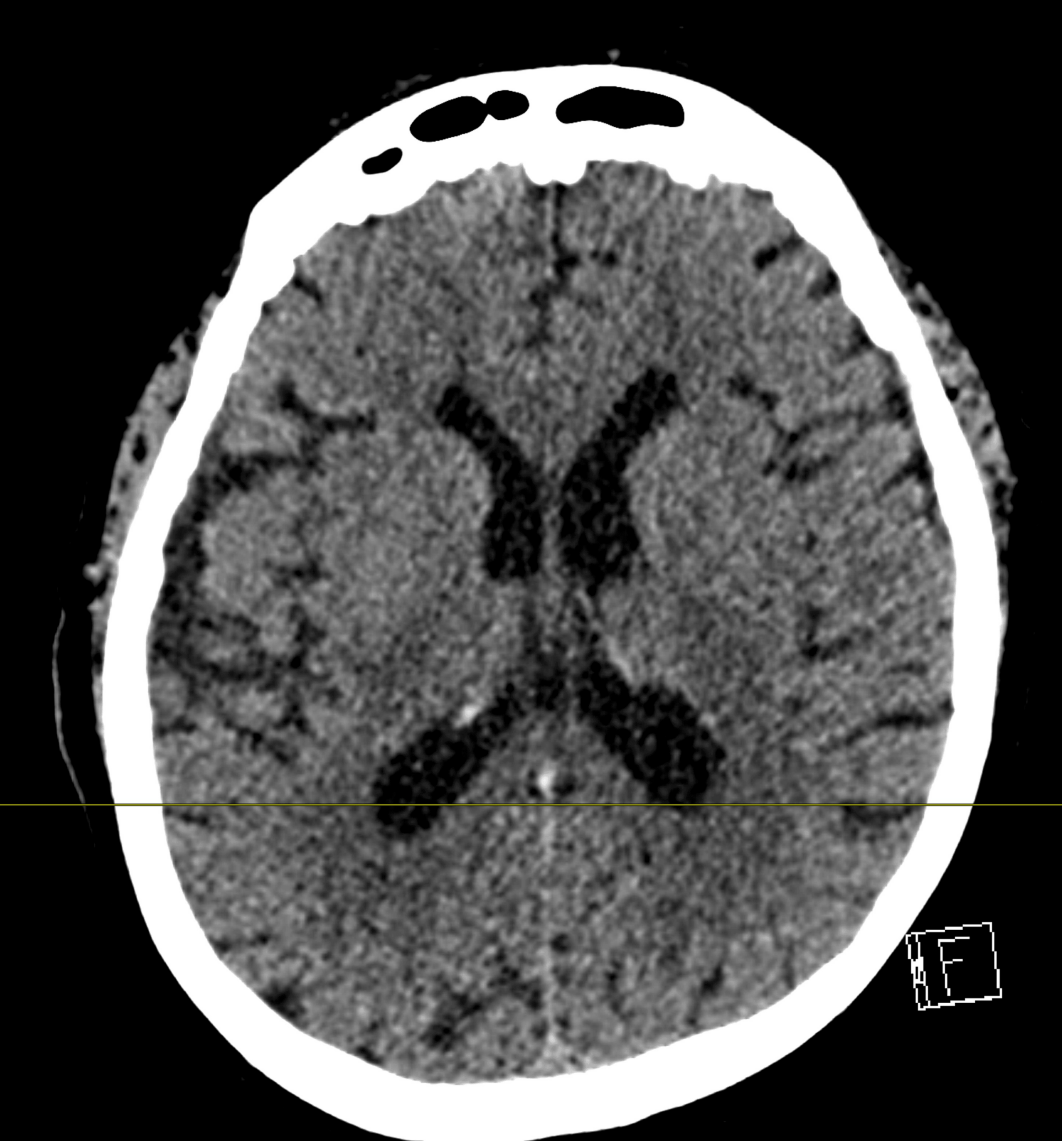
CT of the head Finding: Axial non-contrast CT of the brain showing normal age-appropriate prominence of ventricular system and cortical sulci without evidence of abnormality. Technique: Axial angled images, 120 kVp, 200 mA, 2 mm slice thickness. CT: computed tomography
